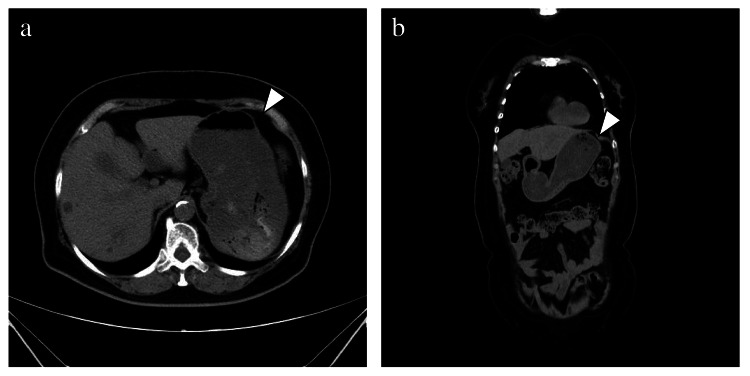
Abdominal computed tomography scan Axial (a) and coronal (b) sections of abdominal CT scan on the arrival to the hospital show a dilated stomach (white arrowhead).
